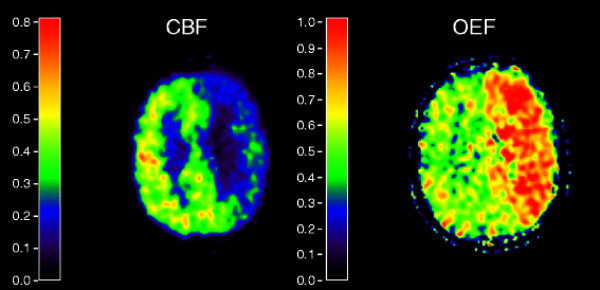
Parametric images of cerebral blood flow and oxygen extraction fraction. Parametric images of cerebral blood flow (CBF) and oxygen extraction fraction (OEF) measured using PET in a 69-year-old man who presented with a minor ischaemic stroke in the left hemisphere with an occlusion of the left internal carotid artery. PET images show decreased CBF and increased OEF in the left hemisphere, indicating haemodynamic failure (stage 2). Using transcranial Doppler, CO_2_-reactivity of the left middle cerebral artery was measured as −12%.
